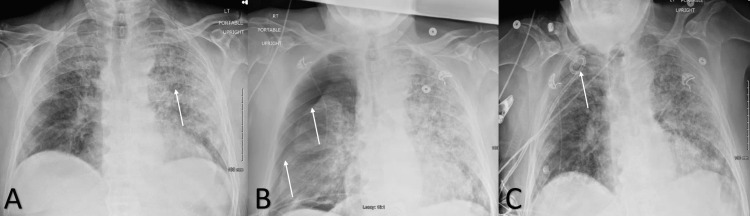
Anterior-Posterior chest x-ray on admission A). Anterior-Posterior chest x-ray on admission showing diffuse ground glass and interstitial opacifications, worse on the left side as indicated by the white arrow. B). Anterior-Posterior chest x-ray showing worsening left-sided ground glass and interstitial opacifications and a right-sided pneumothorax as shown by the white arrows. C). Anterior-Posterior chest x-ray with resolution of right-sided pneumothorax after placement of a 14 French pigtail catheter, as shown by the white arrow in the apex of the chest.
